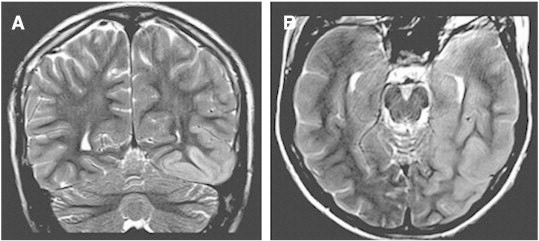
MRI of brain. There is cortical thickening with mildly associated increased T2 signal within the left posterior temporal and mesotemporal lobe with involvement of the hippocampal and parahippocampal gyri. A. Coronal T2-weighted image; B. axial T2-weighted image.
